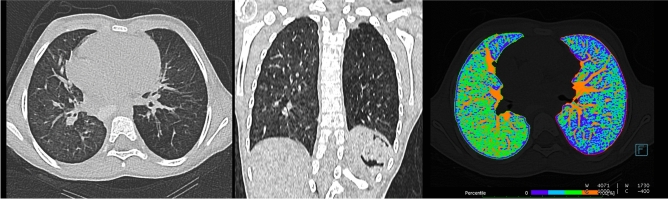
CT data of 10 year old child after CDH repair. Child after left sided CDH repair. The visual evaluation of the images already reveals lower density values on the ipsilateral/left side. On the right: Colour coded image of lung density (green shows normal lung density, blue codes hypoattenuated lung parenchyma).
